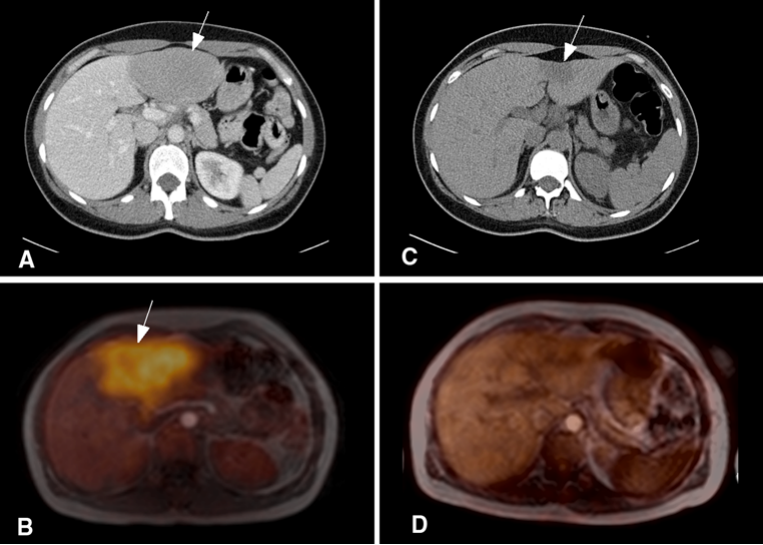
FDG-PET/MR and corresponding CT images of a patient with diffuse large B cell lymphoma. a Contrast enhanced CT scan showing a hepatic lymphoma manifestation before chemotherapy. b Fused FDG-PET/MR before chemotherapy. c Nonenhanced CT scan after chemotherapy showing a residual mass (*arrow*). d Corresponding PET/MR after chemotherapy showing no pathologic FDG uptake
